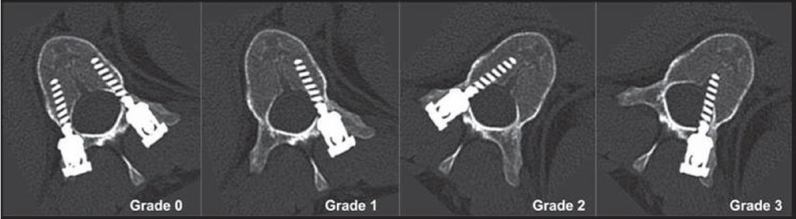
Grading used for pedicle breaches on postoperative CT scan images
